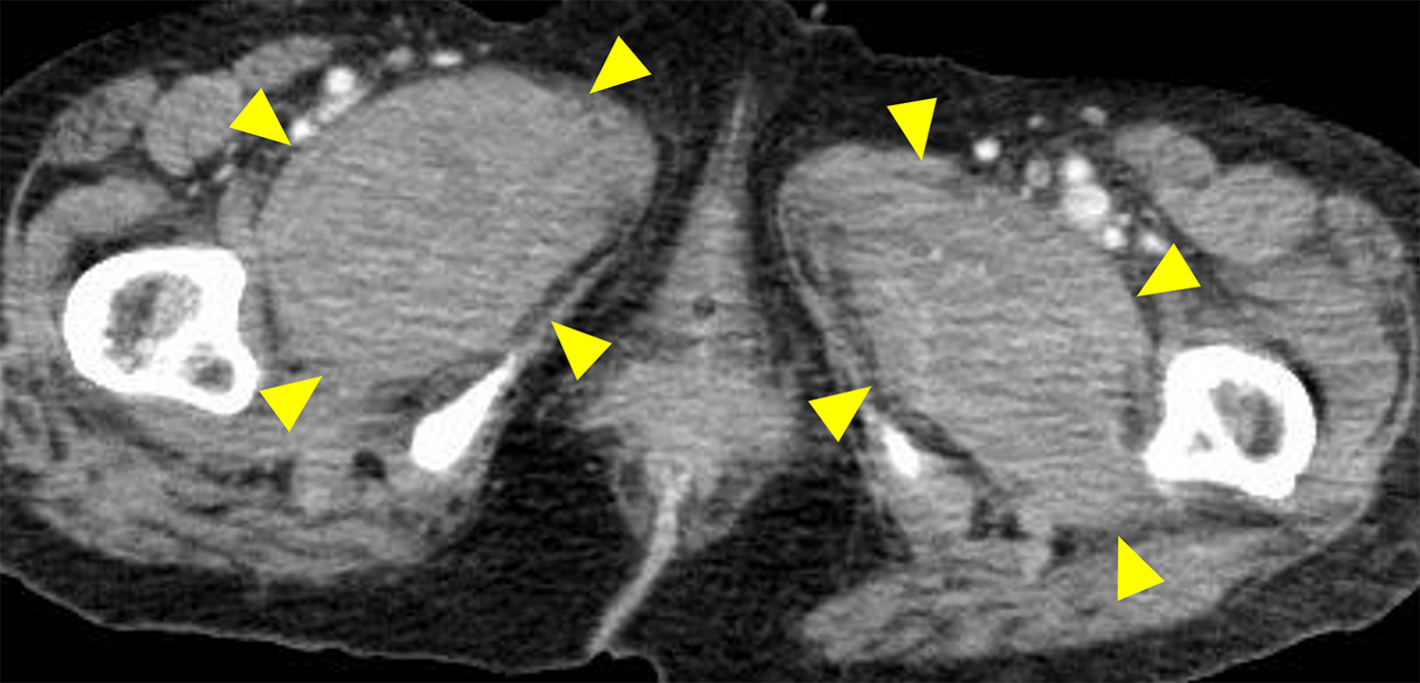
Contrast-enhanced computed tomography showing hemorrhages within bilateral adductor muscles (arrowheads).
